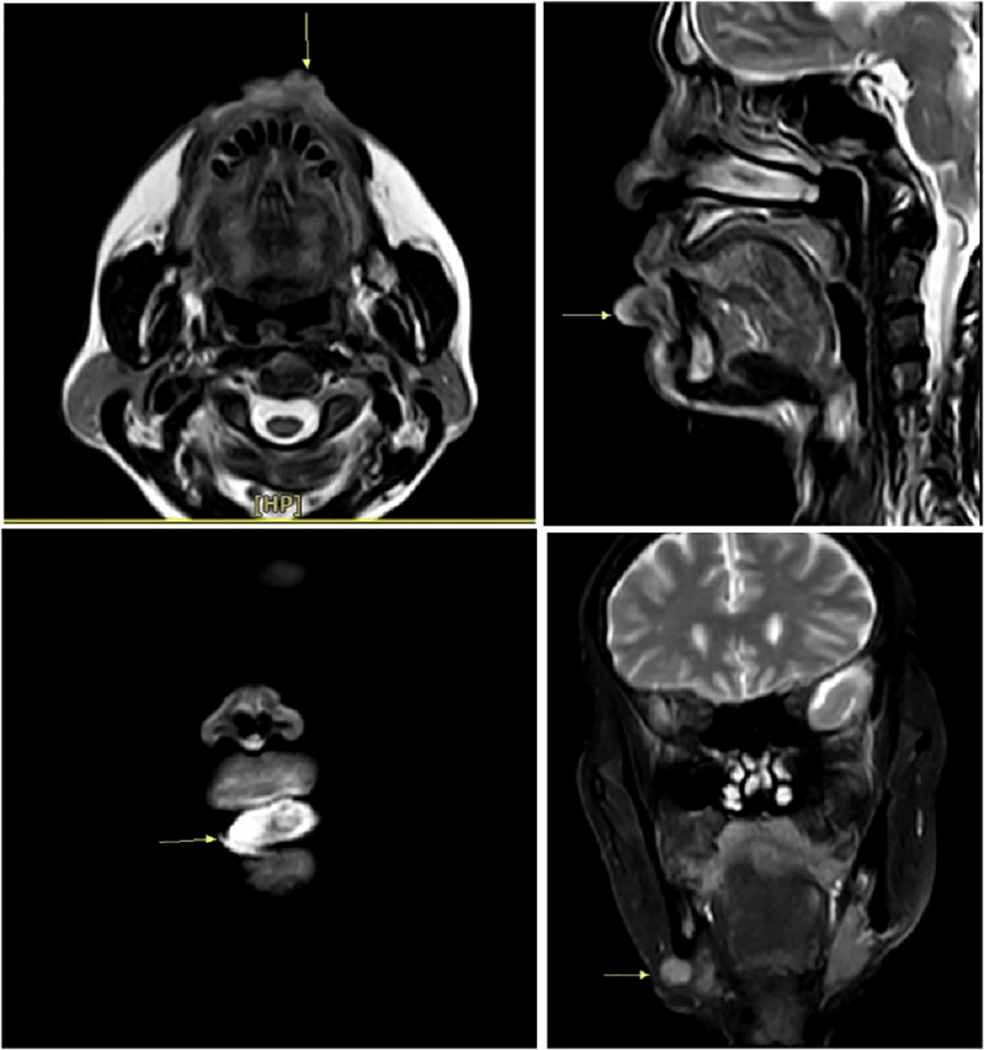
CECT of squamous cell carcinoma of the lip; the arrow indicates the site of the lesion top left: axial; top right: sagittal; bottom: coronal images CECT: contrast-enhanced computed tomography
